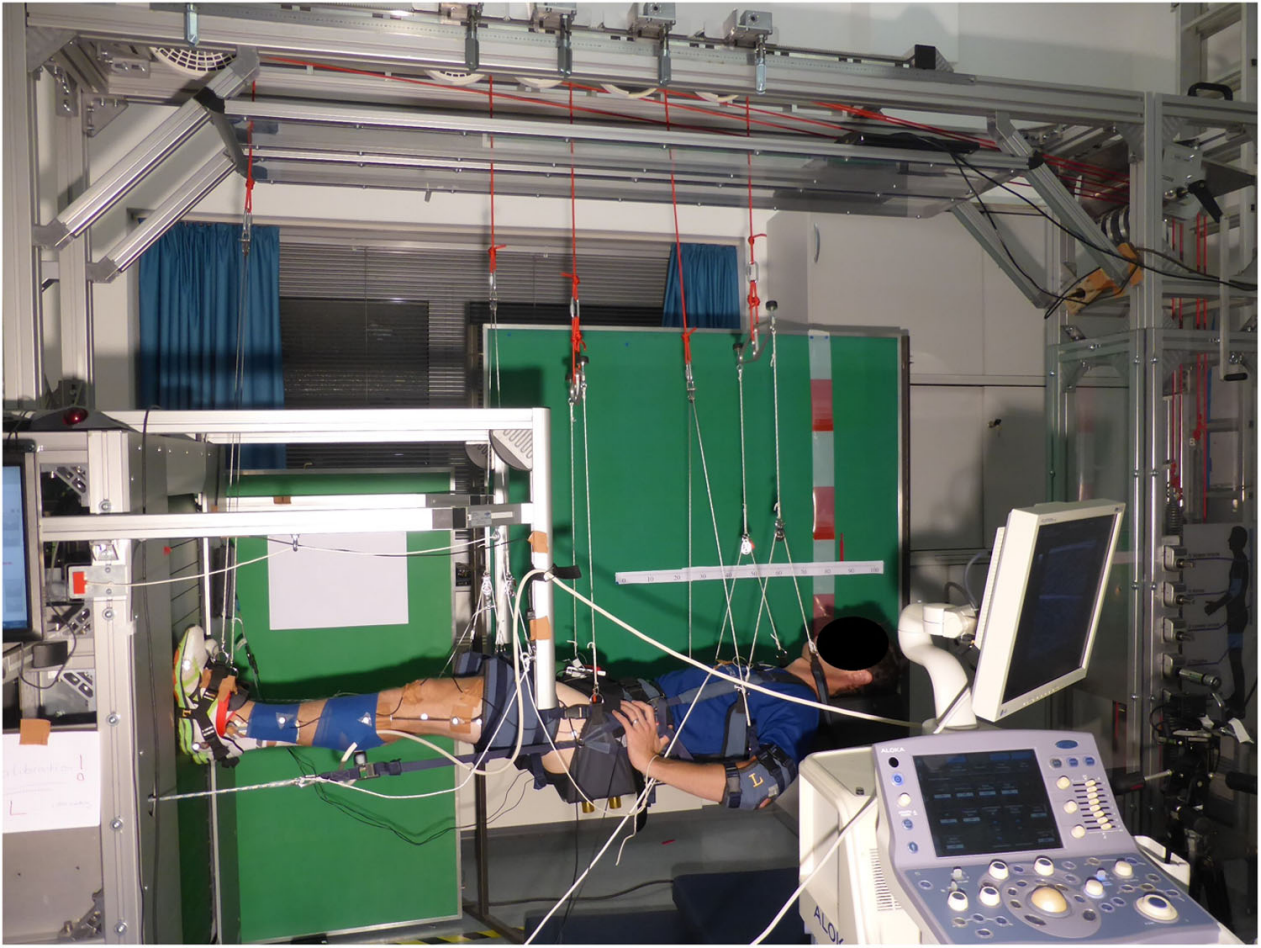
VTF experimental set-up. Participant being suspended horizontally on the vertical treadmill facility (VTF) with an ultrasound transducer attached to the mid-belly of the GM muscle and electrogoniometers to record knee and ankle joint angles. Photo credit Charlotte Richter; participant provided written informed consent to publish this photo.
